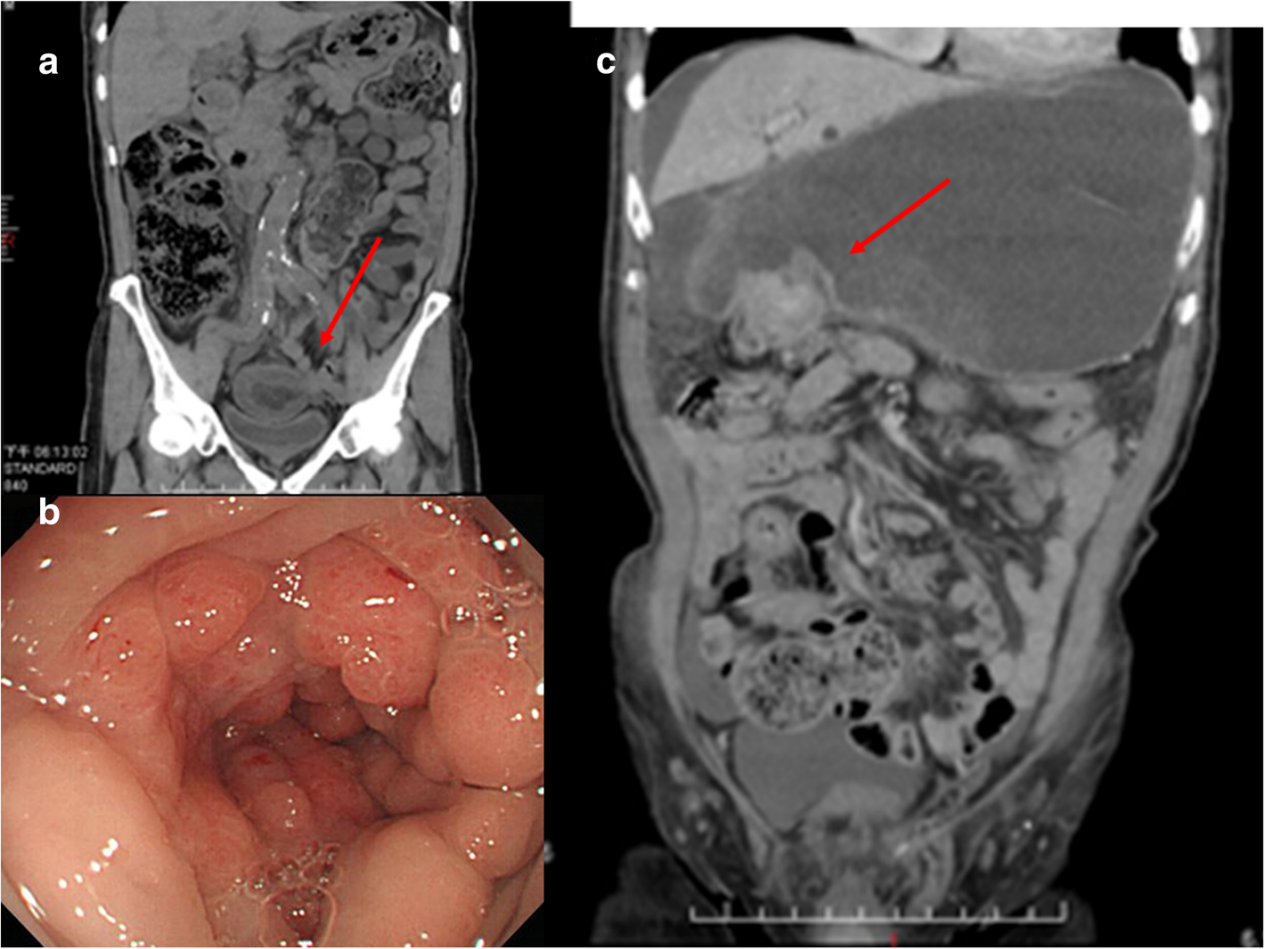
Synchronous colonic metastasis of advanced GC. a Abdominal CT showed suspected rectosigmoid colon cancer with partial obstruction; b colonoscopy revealed rectosigmoid colon wall thickening and partially obstructed mucosal swelling; c abdominal CT displayed circumferential thickening of the pylorus and marked stomach distension, indicating the possibility of GC
